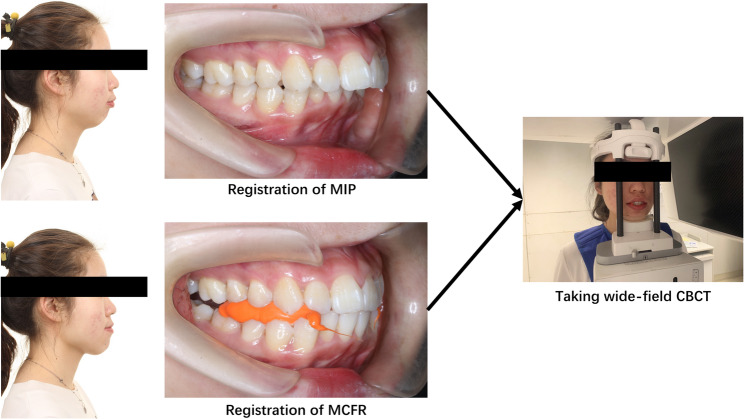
After registration of MIP and MCFR, patients underwent wide-field CBCT
